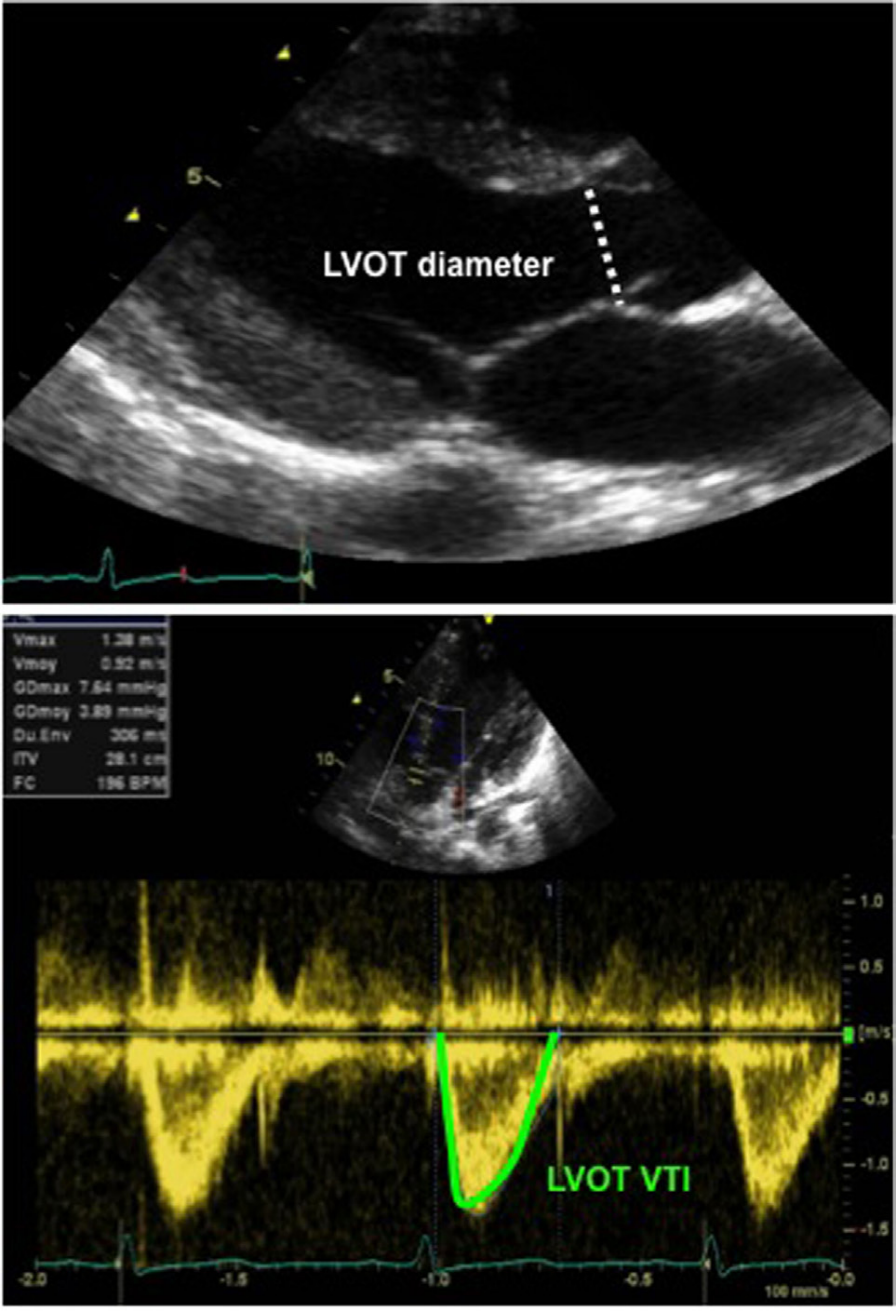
Echocardiographic assessment of cardiac output: 2D echocardiography long-axis view with measurement of LVOT diameter (above image), allowing for calculation of the LVOT CSA and Doppler tracing from an apical five-chamber view (below image) with tracing of the LVOT velocity to obtain the LVOT VTI. Abbreviations: CSA, cross-sectional area (calculated as π × *r*^2^ and *r*, radius, diameter/2): LVOT, left ventricular outflow tract; VTI, velocity time integral (or mean velocity).
